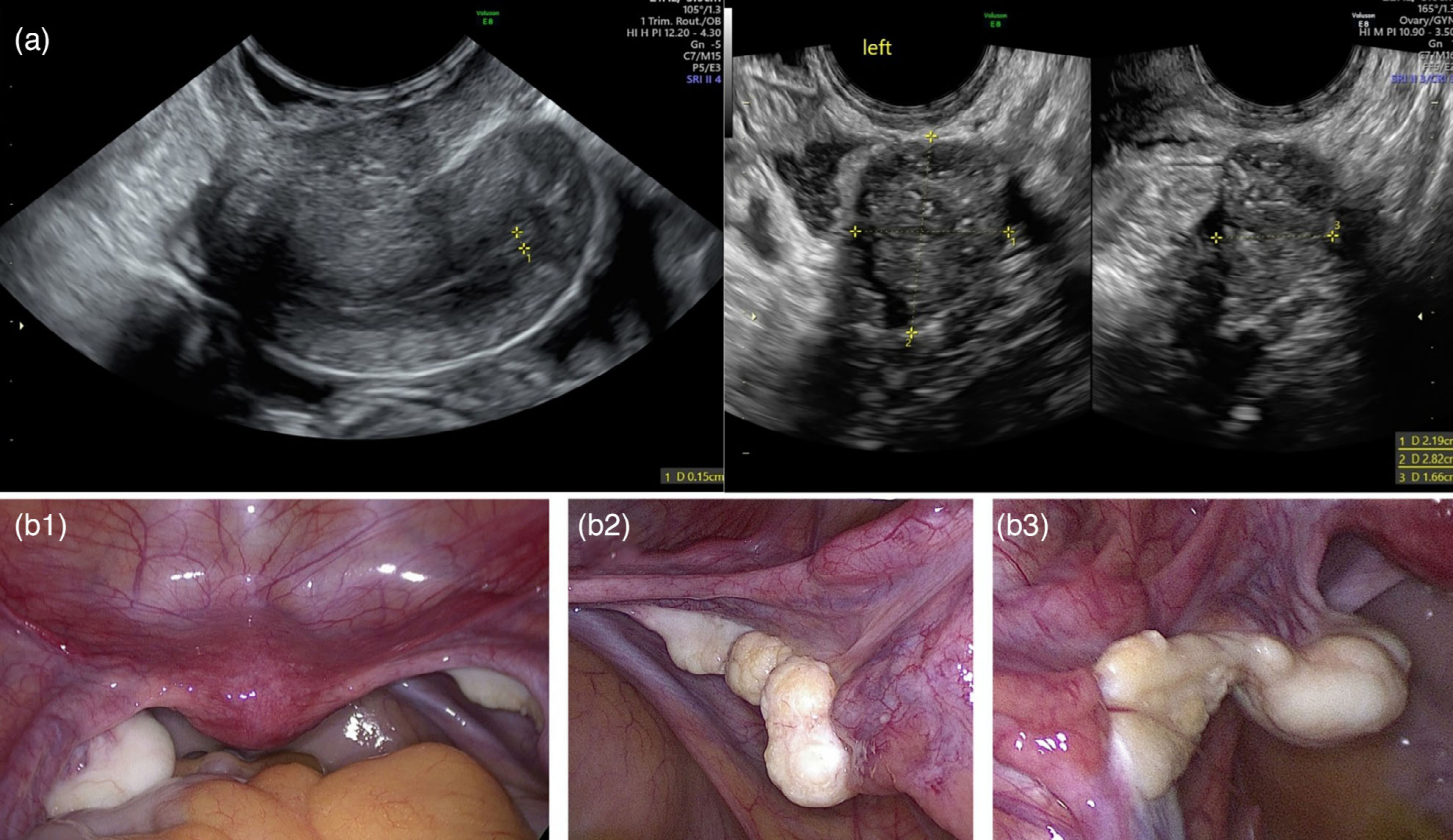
(a) Pelvic ultrasound showing a hypoplastic uterus measuring 42 × 18 × 29 mm with thin endometrium (on the left) and the left adnexa revealing a nonfunctional ovary with hyperechoic foci suggestive of calcifications (on the right). (b) 1. Laparoscopic view of the uterus and adnexa; 2. right adnexa; 3. left adnexa.
